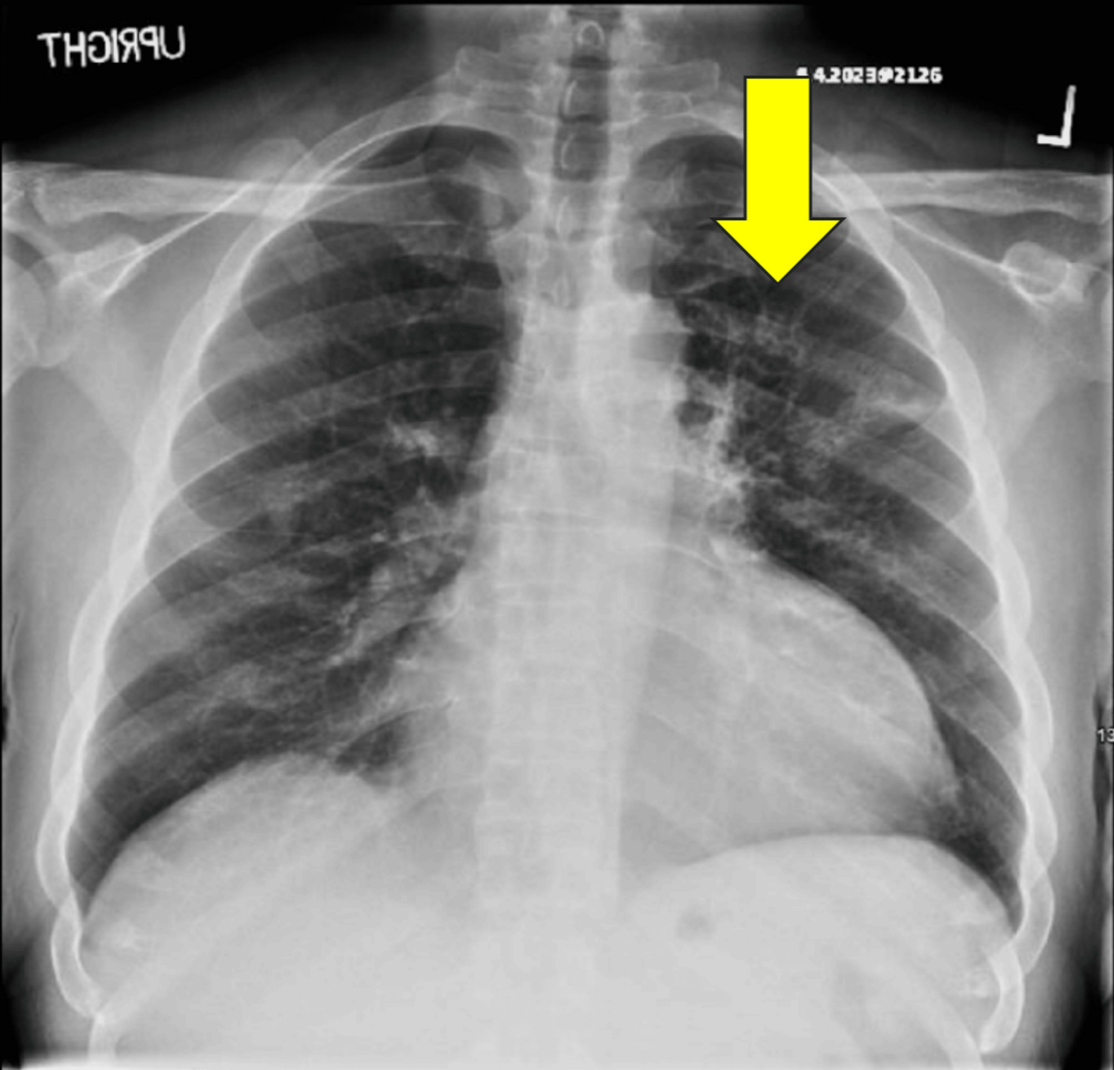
Anteroposterior view of chest X-ray demonstrating patchy patchy airspace disease in the left upper lobe.
